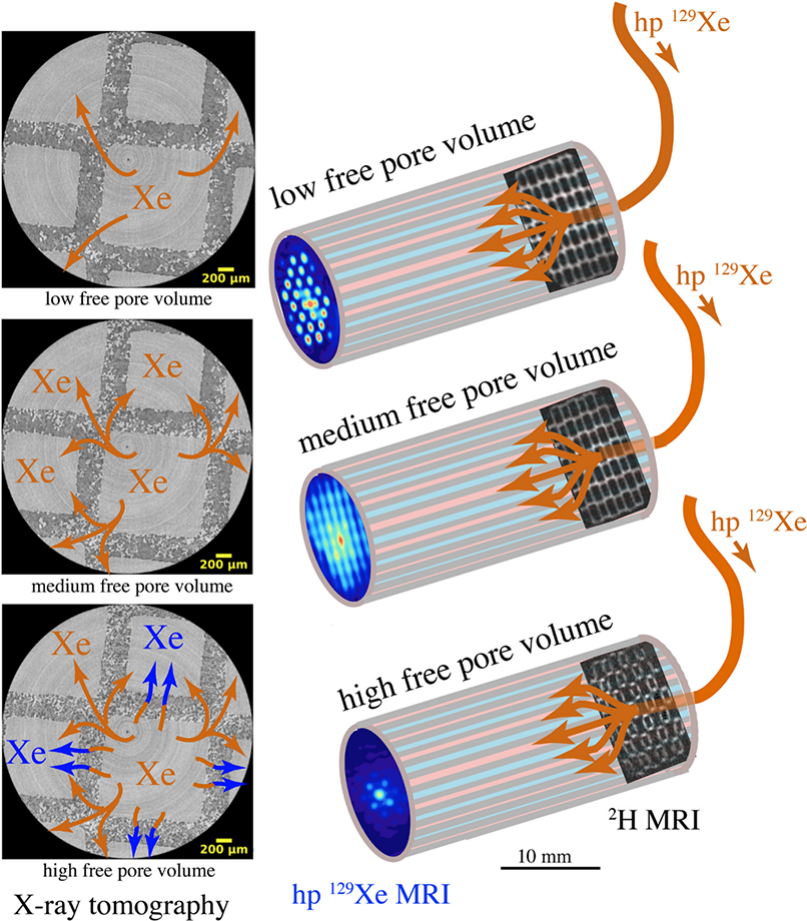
Hyperpolarized ^129^Xe MRI, ^2^H MRI, and X-ray
